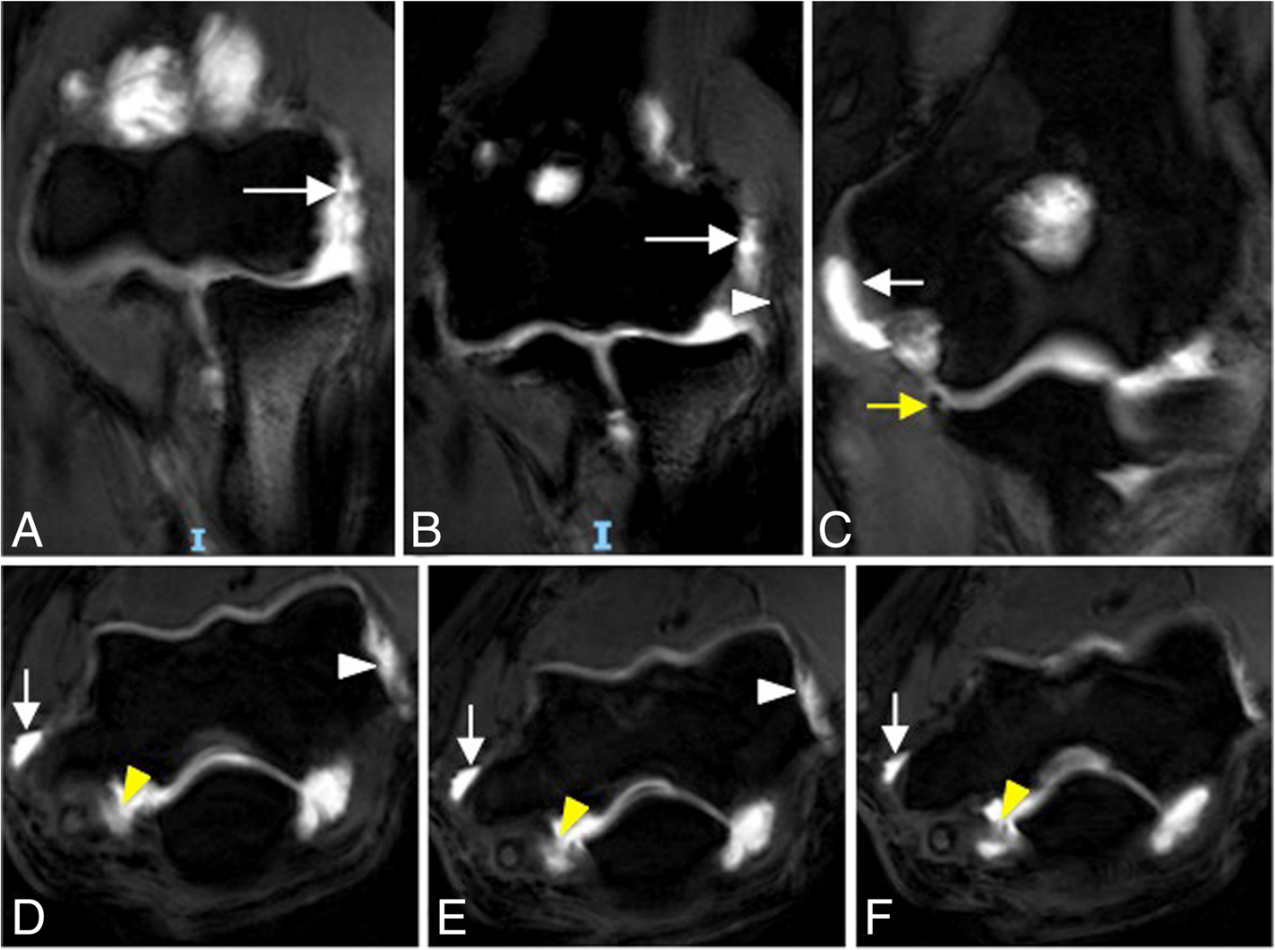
Posterolateral rotatory instability, stage 3C. Consecutive coronal FS T1-weighted direct MR arthrographic images (a–c), and consecutive axial FS T1-weighted direct MR arthrographic images (d–f) demonstrating a full-thickness tear of the anterior bundle of the medial collateral ligament complex (yellow arrow), proximal full-thickness tear of the common flexor tendon (short white arrows), proximal full-thickness tear of the common extensor tendon (white arrowheads), proximal complete tear of the lateral ulnar collateral ligament and radial collateral ligament proper (long white arrows), and a complete tear of the posterior bundle of the medial collateral ligament complex (yellow arrowheads)
